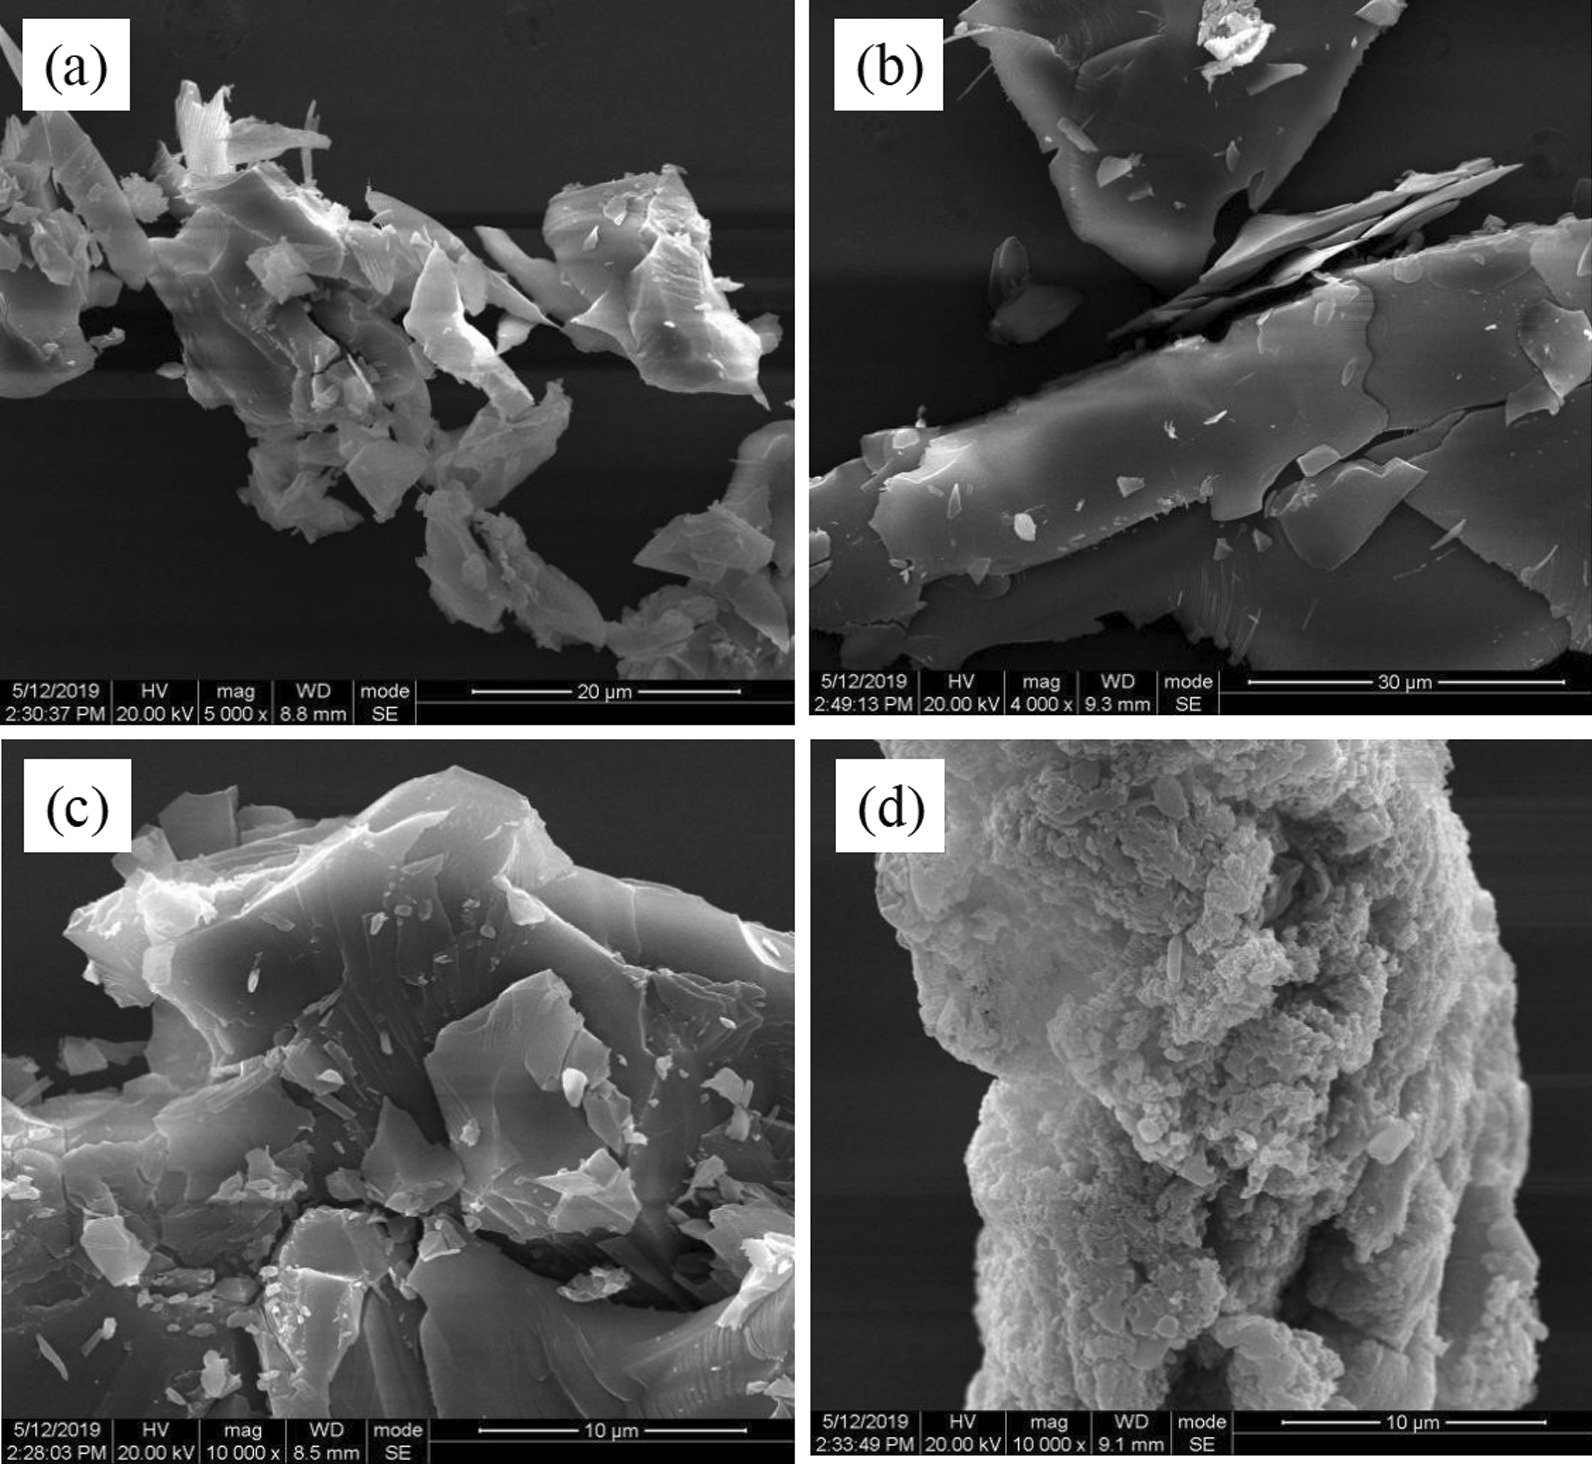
SEM images of MoS_2_ nanosheets under different ultrasonic power. The ultrasonic powers are a 240 w, b 360 w, c 480 w, d 600 w, respectively
